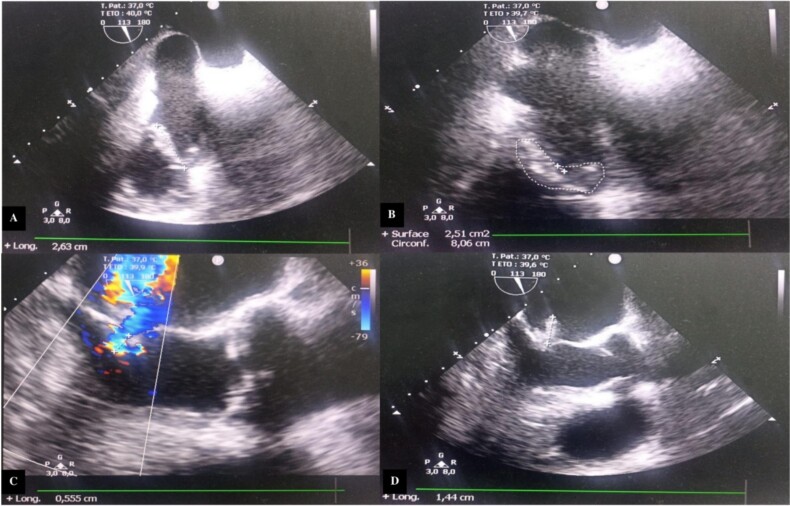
Transesophageal echocardiography images. (A) Incidence 113° showing vegetation attached to the free edge of the anterior tricuspid leaflet measuring 26.3 mm in long axis. (B) Surface area of the vegetation attached to the anterior tricuspid leaflet estimated at 2.51 cm^2^ and circumference of 8.06 cm. (C) Color Doppler showing significant mitral regurgitation. (D) Incidence 113° showing vegetation attached to the large mitral leaflet measuring 15 mm in long axis.
